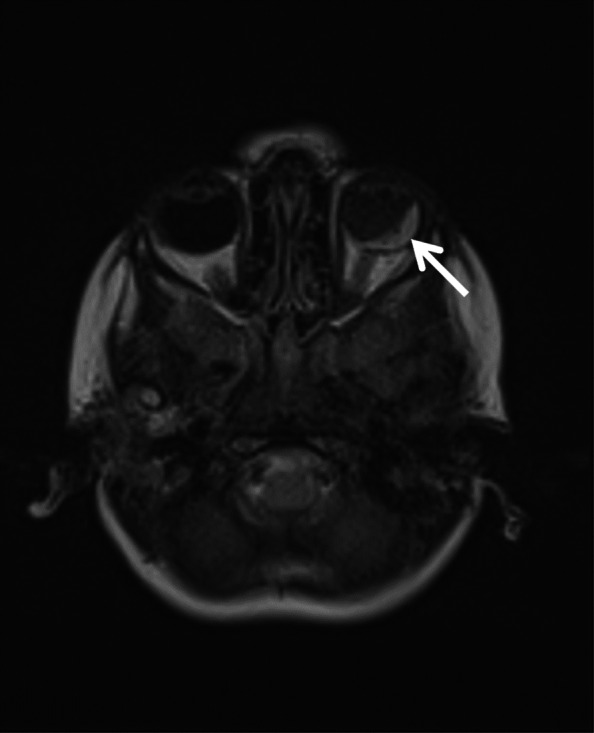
Hemorrhage in left eye in cranial MRI
